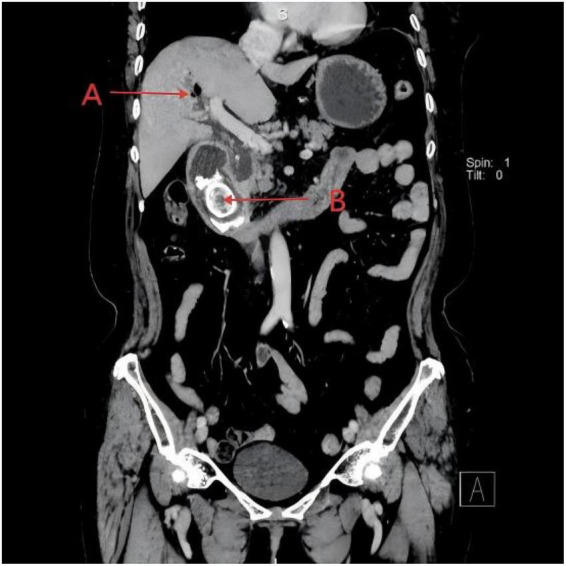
Coronal abdominal computed tomography (CT) showing a giant gallstone impacted in the descending duodenum, consistent with imaging features of Bouveret syndrome. Arrow A indicates pneumobilia. Arrow B indicates ectopic giant gallstone in the duodenum.
